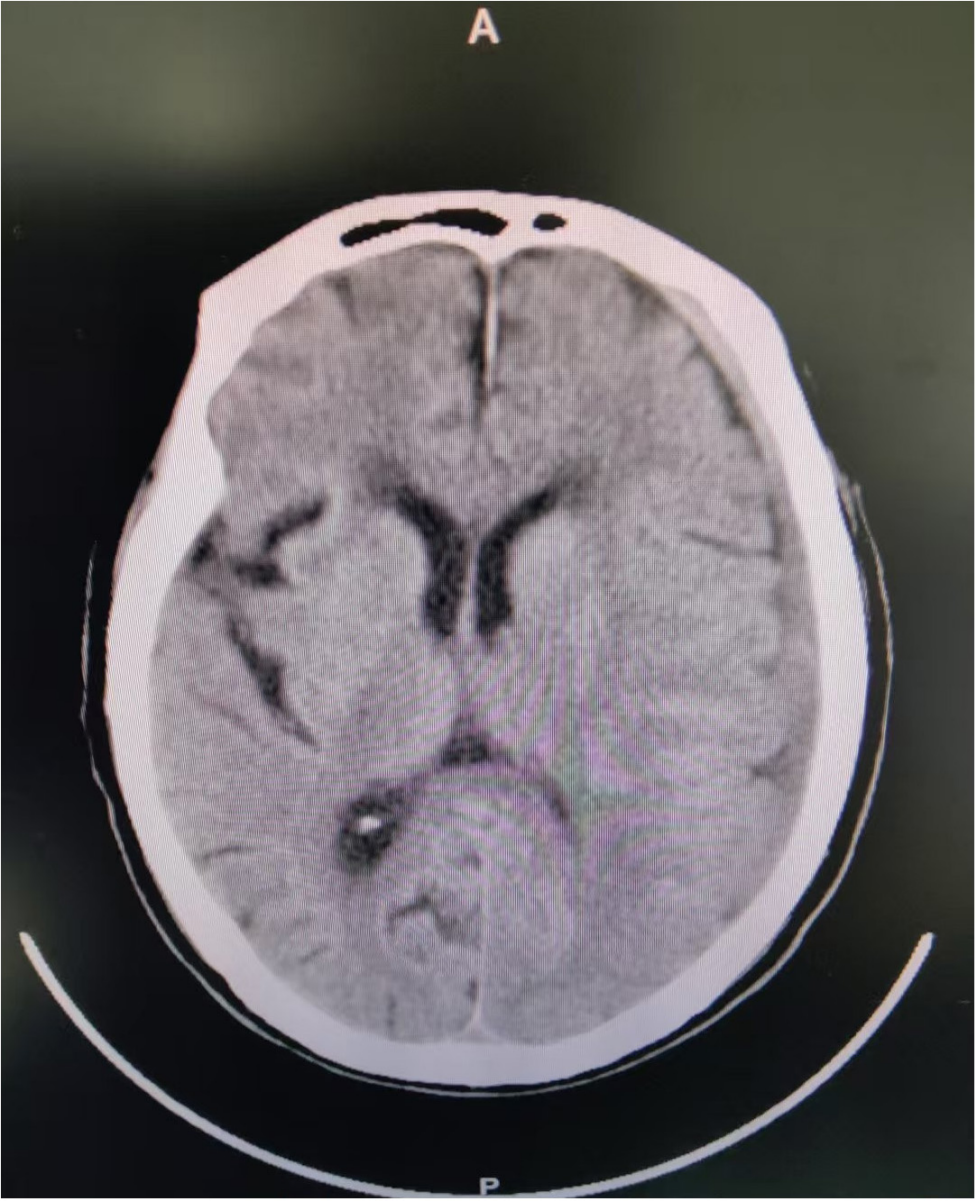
The patient’s brain CT examination after the car accident showed a small left subdural hematoma.
